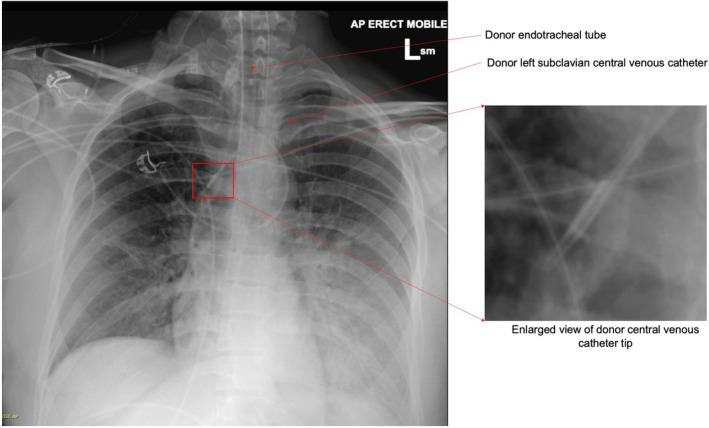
Chest radiograph of the donor before organ procurement with a left subclavian CVC in situ.
